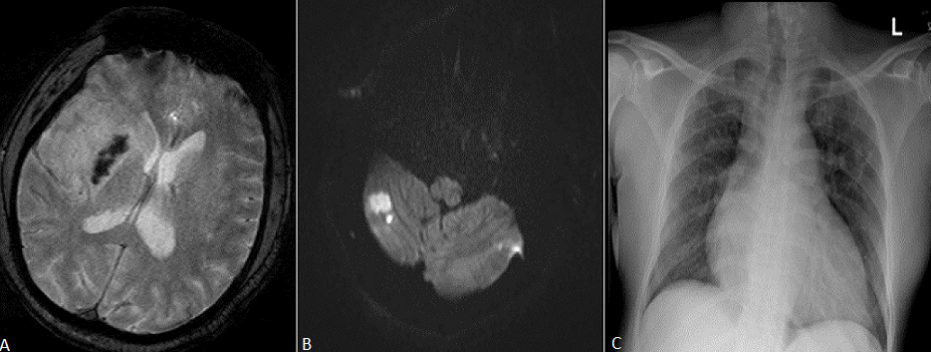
(A) GRE Haem sequence demonstrates low signal intensity in the region of right basal ganglia representing haemorrhagic transformation; (B) MRI DWI shows multiple hyperintense areas of restricted diffusion in the cerebellum in keeping with acute infarctions; (C): chest X-ray, AP reveals poorly defined infiltrates in the right upper zones suggestive of pneumonic changes
